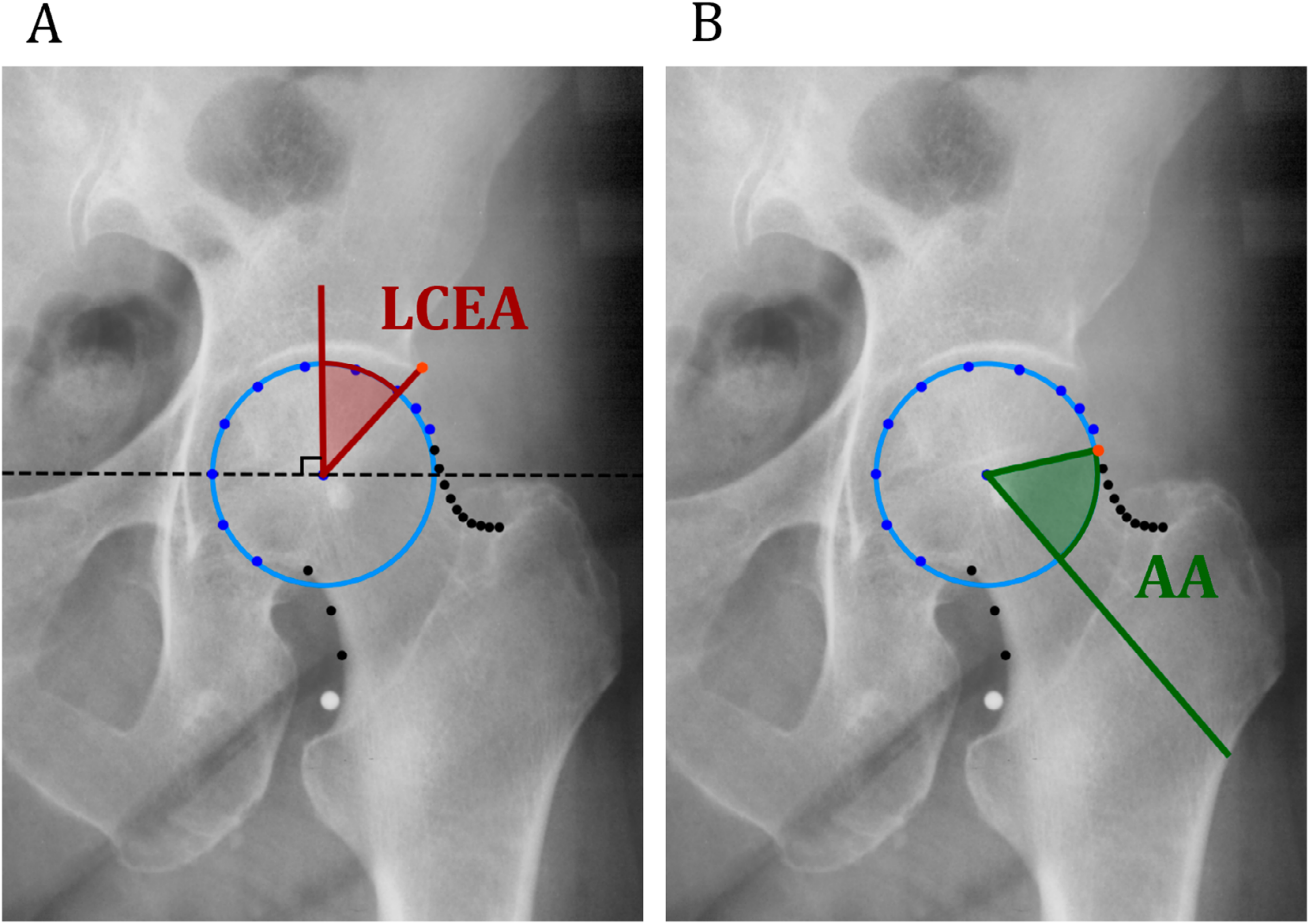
Anteroposterior pelvic radiographs focused on the left hip showing the automatically measured morphology measurements. These methods rely on automated landmark points. To determine the center of the femoral head, a best‐fitting circle (light blue) is outlined around the femoral head based on a subset of femoral head landmark points (dark blue). (A) LCEA (in red): The angle is calculated between a line drawn vertically through the center of the femoral head, perpendicular to the horizontal reference line (black, dashed), and a line originating from the center of the femoral head to the most lateral part of the acetabulum (orange landmark). (B) AA (in green): The angle is calculated between a line through the femoral head center and the point where the femoral head or femoral neck is first outside of the best‐fitting circle (orange landmark) and the femoral neck axis. AA, alpha angle; LCEA, lateral center edge angle.
